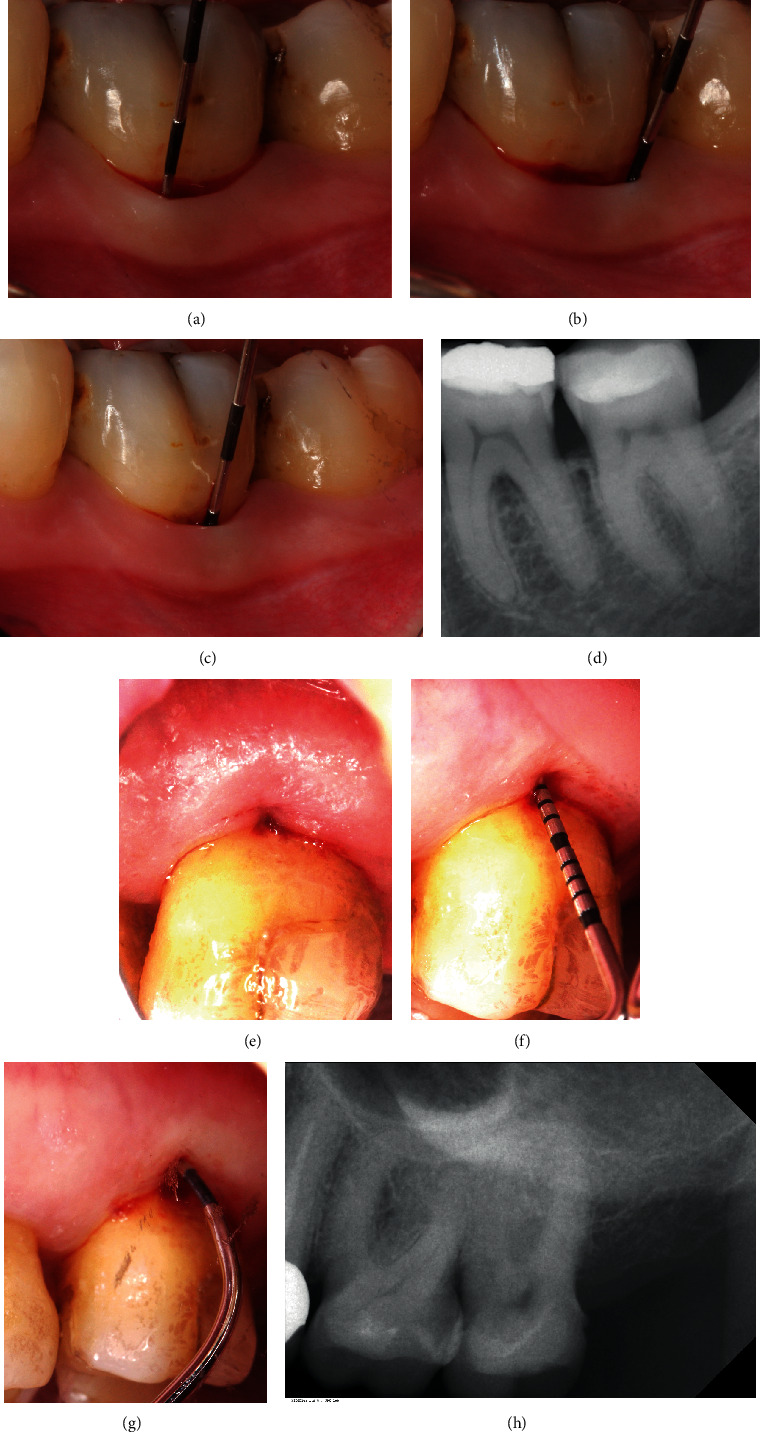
(a) Tooth 36 (FDI) baseline mesiobuccal VPD with 3 mm PPD F. (b) Tooth 36 (FDI) baseline distobuccal VPD with 3 mm PPD. (c) Tooth 36 (FDI) baseline 5 mm buccal VPD at the furcation entrance. (d) Tooth 36 (FDI) baseline periapical X-ray with furcation involvement grade 2. (e) Tooth 26 (FDI) baseline image of the gingiva margin at the furcation area. (f) Tooth 26 (FDI) baseline buccal 6 mm VPD at the furcation entrance. (g) Tooth 26 (FDI) baseline 6 mm HPD indicating buccal FI grade 2. (h) Tooth 26 (FDI) baseline periapical X-ray with furcation involvement grade 2.
